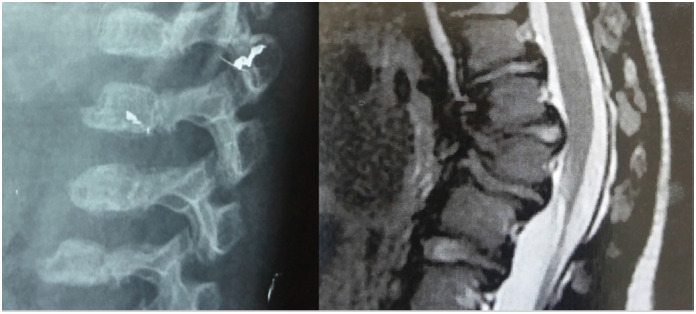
In this figure, it is possible to see the typical shape of the vertebrae (betta fish) on the X-ray, as well as the retropulsion of the intervertebral discs causing stenosis of the spinal canal at the kyphosis apical vertebra.
